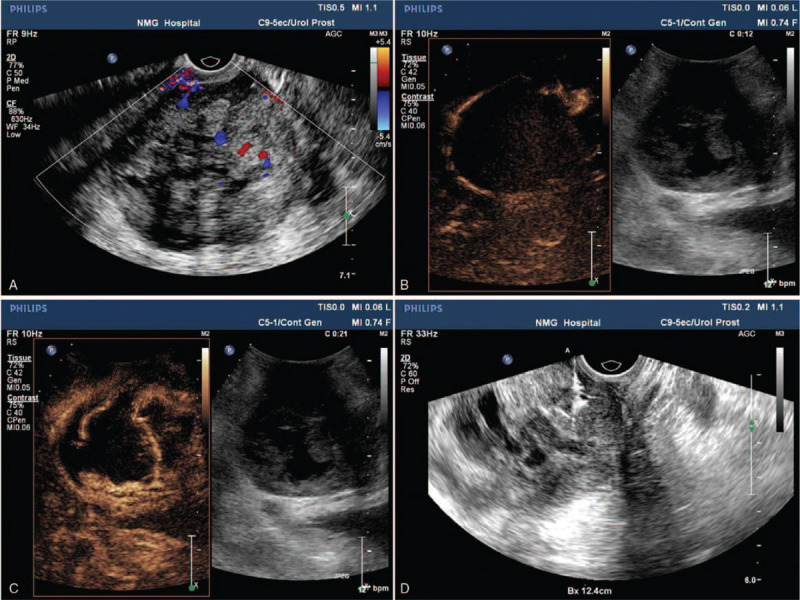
US imaging findings of the PS. Conventional US reveals a multiloculated soft tissue mass in the prostate within rich blood supply signals from the surrounding soft tissue (A). CEUS shows that the peritumoral parenchyma began to enhance rapidly in 12 s (B) and large blood vessels in the necrosis liquefying area of the tumor center (C). According to the enhanced area shown by CEUS, a biopsy was performed (D). CEUS = contrast-enhanced ultrasonography, PS = prostatic sarcoma, US = ultrasonography.
